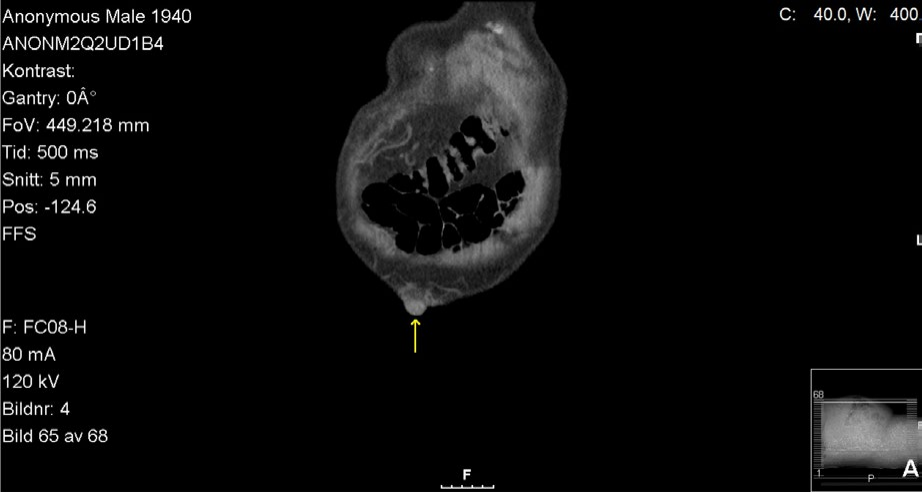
CT without contrast (coronal) shows dilated paraumbilical veins
